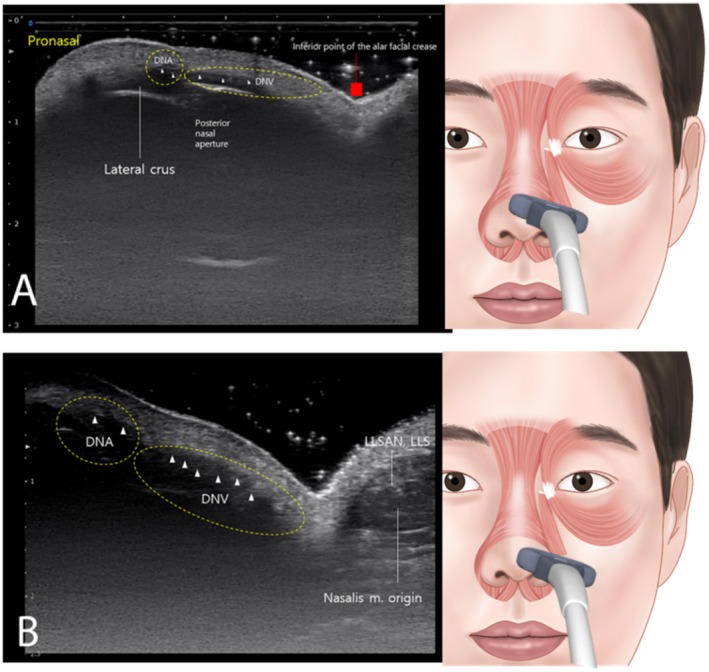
The ultrasound observations obtained through probe placement in specific nasal regions. In panel A, placing the probe diagonally at the pronasal area shows the presence of the lateral crus and the dilator naris anterior (DNA), and dilator naris vestibularis (DNV), originating from the posterior nasal aperture and extending vertically to the dermal layer. Additionally, the alar groove acts as a demarcation line separating the DNA and DNV. Panel B displays that the nasalis muscle, consisting of the ala and the transverse parts, originates from the maxilla and intertwines with the levator labii superioris alaeque nasi (LLSAN) and levator labii superioris (LLS) (B mode, 18‐MHz linear transducer).
